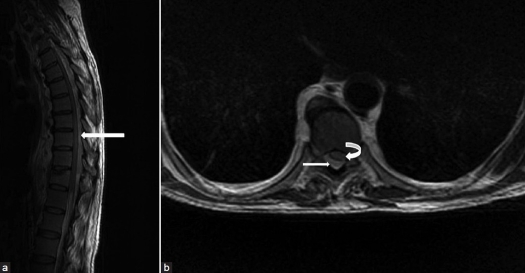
(a) T2-weighted sagittal magnetic resonance image of the thoracolumbar spine showing the isointense extradural lesion (arrow) causing thecal sac and spinal cord compression and signal intensity changes within the cord. (b) The T2-weighted axial image showing thecal and spinal cord compression (curved arrow) by the extradural lesion (straight arrow)
